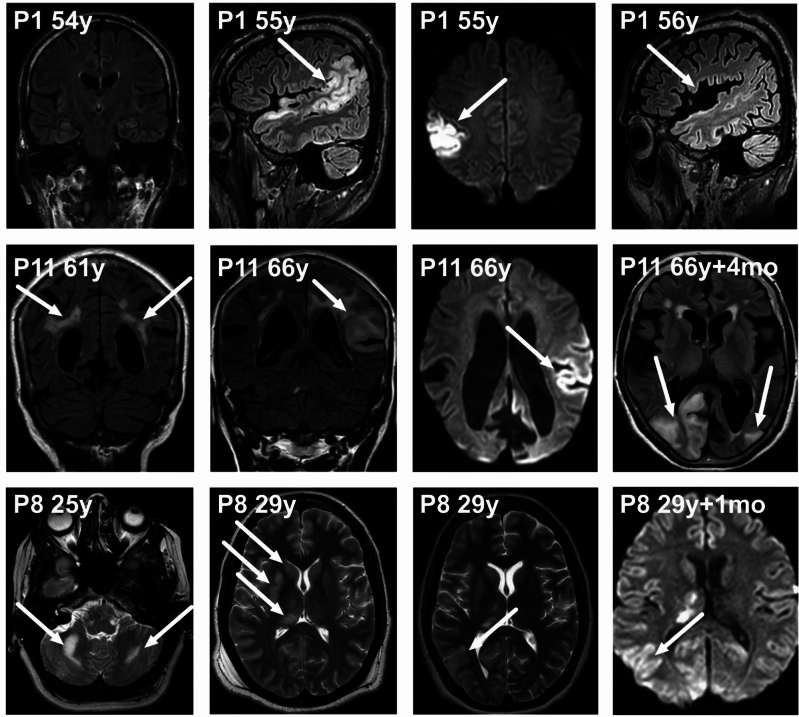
Brain MR imaging findings in patients with stroke-like episodes (SLEs)
